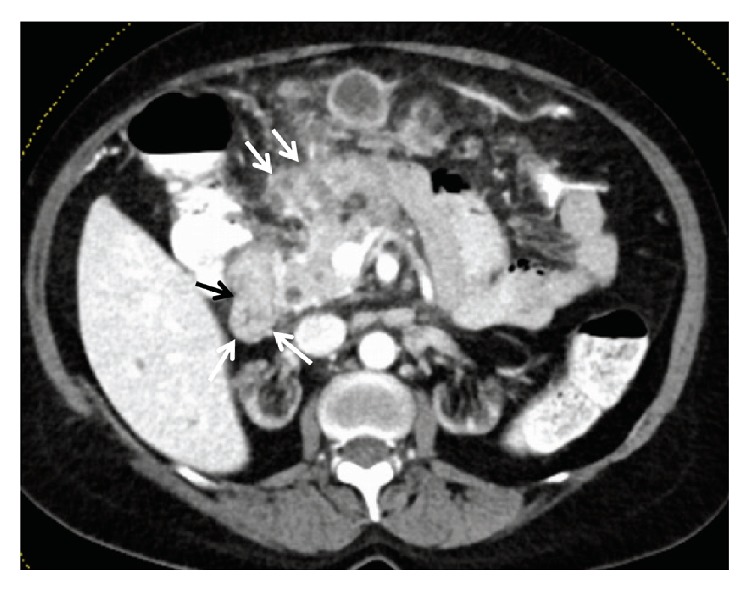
CT scan with multiple contrast-enhancing peritoneal nodules and large intraperitoneal soft-tissue masses (arrows), radiologically impressing as severe peritoneal carcinomatosis.
